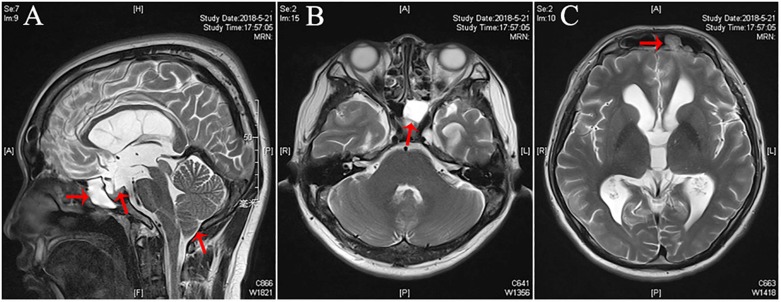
Pre-operative imaging of the patient with CSF rhinorrhea. (A) Pre-operative sagittal T2-weighted magnetic resonance imaging (MRI) demonstrating hyperintense in the left sphenoidal sinus, partial empty sella, and Chiari I malformation (CIM) (arrows). (B) Pre-operative axial T2-weighted MRI demonstrating hyperintense in the left sphenoidal sinus (arrows). (C) Pre-operative axial T2-weighted MRI demonstrating a left sided meningoencephalocele (arrow) extending into the left frontal sinus.
